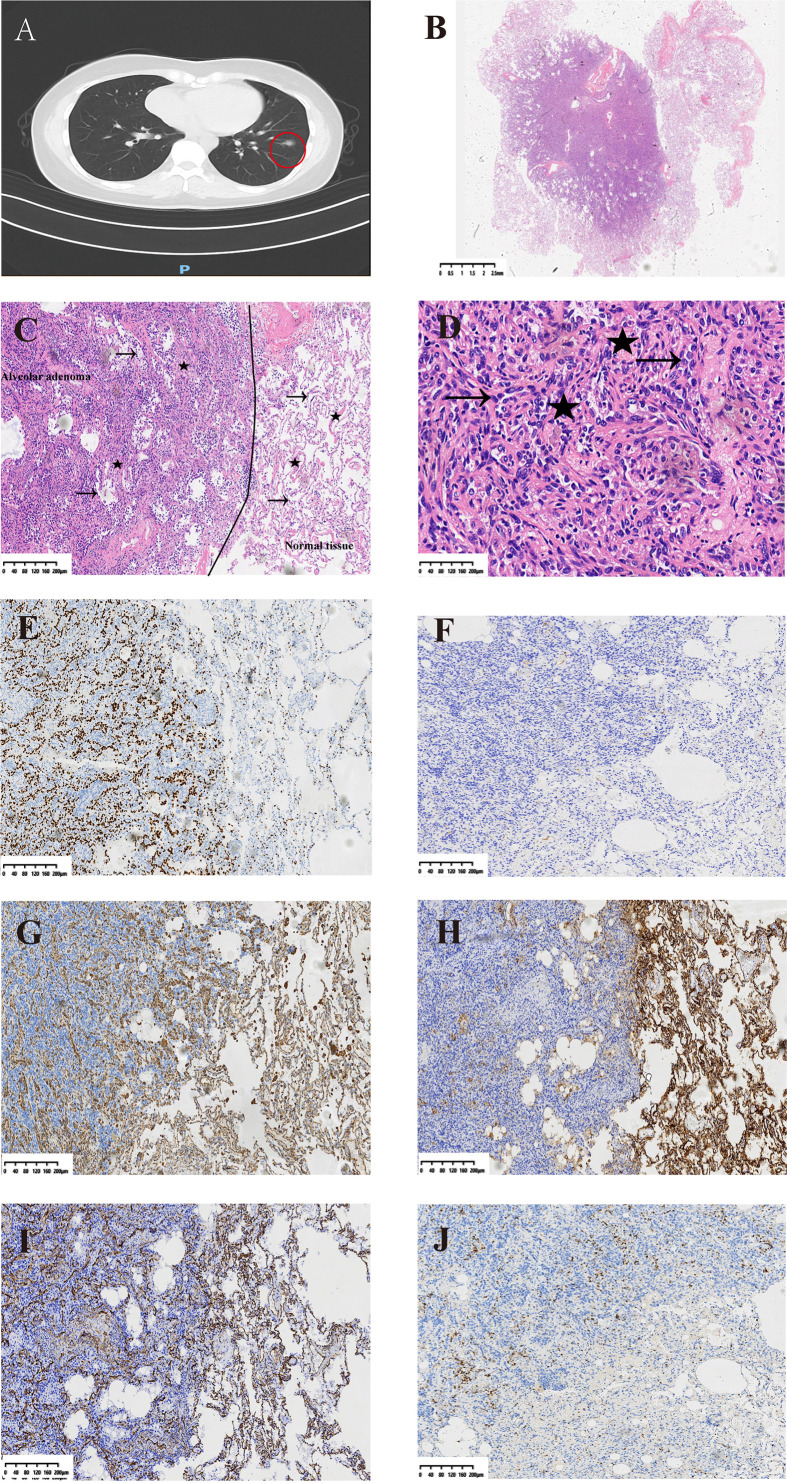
(A) CT scan showing a well-defined solid nodule; (B) The tumor is distinctly separated from adjacent lung tissue (10x). (C) The tumor is made up of densely packed glandular epithelium (arrows), partially arranged in fissure-like patterns, surrounded by proliferating stromal cells (asterisks) (100x). (D) Most epithelial cells (arrows) are arranged in a single layer, with no significant atypia in either epithelial or stromal cells (asterisks) (400x). (E) Expression of TTF-1 (100x); (F) Expression of P40 (100x). (G) Expression of vimentin (100x); (H) Expression of CD10 (100x); (I) Expression of CD34 (100x). (J) Expression of BCL-2 (100x).
